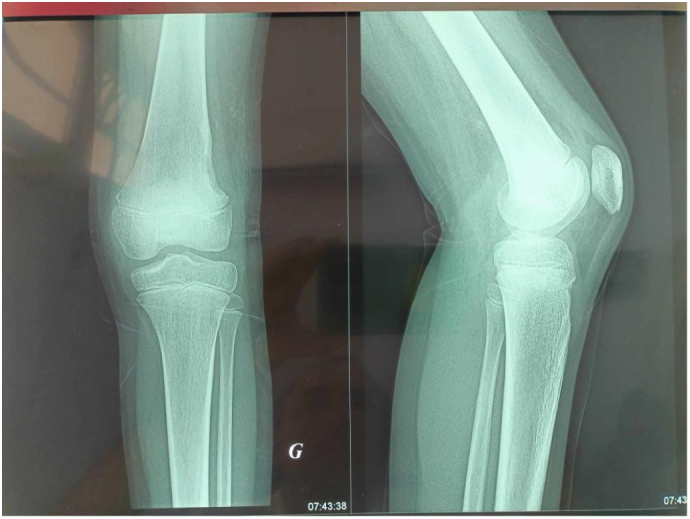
Anteroposterior and lateral radiographs of the knee reveal a well-defined osteolytic lesion measuring 5 cm, located in the external cortex of the distal femoral metaphysis. The lesion exhibits a sclerotic margin, a narrow zone of transition, and no evidence of periosteal reaction.
